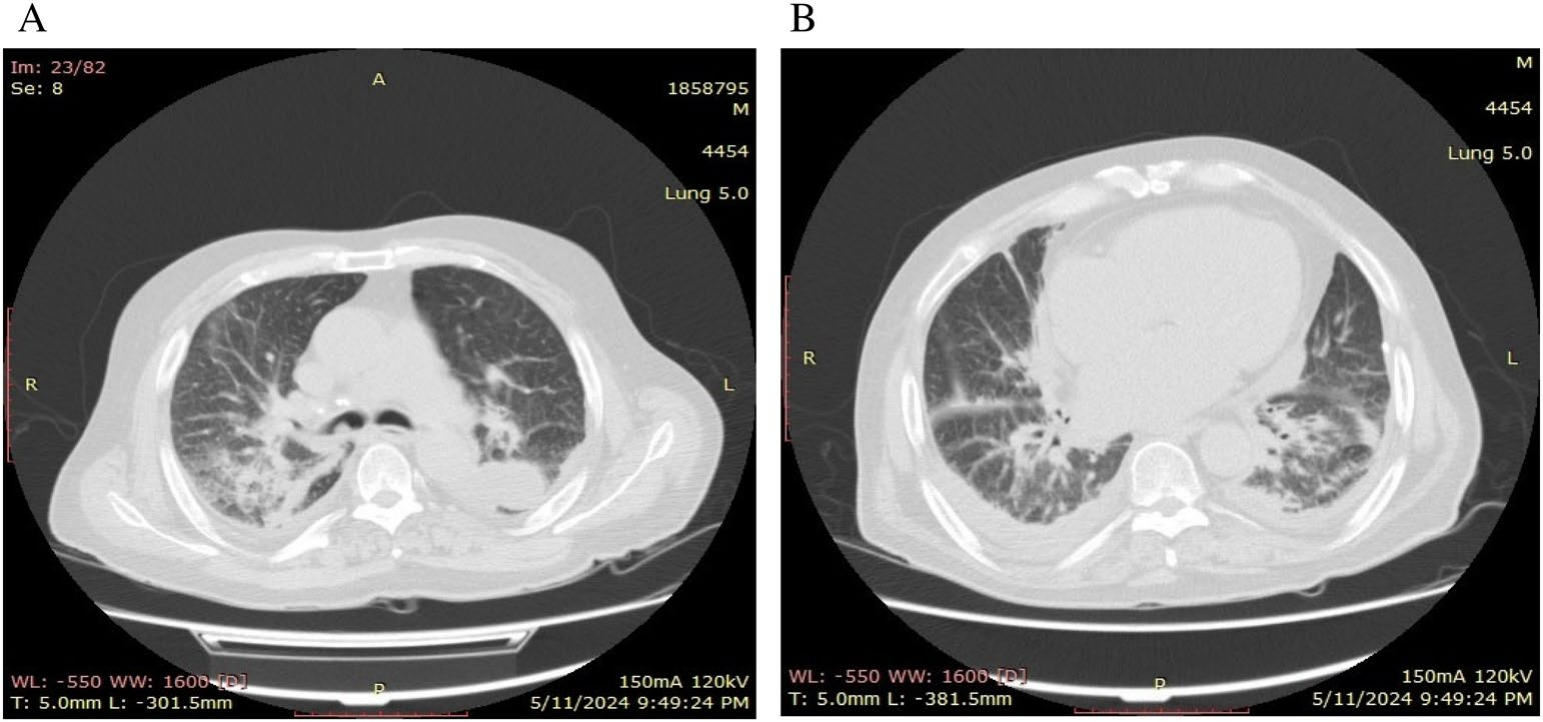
(A) A chest computed tomography scan (CT scan) demonstrated multiple calcified mediastinal and hilar nodules, calcified granulomas in both lungs, and air‐space opacification. (B) A chest computed tomography scan (CT scan) demonstrated an increase in the thickness of the interlobular septum, bilateral pleural effusions, and accumulation of fluid in the fissure of both sides.
